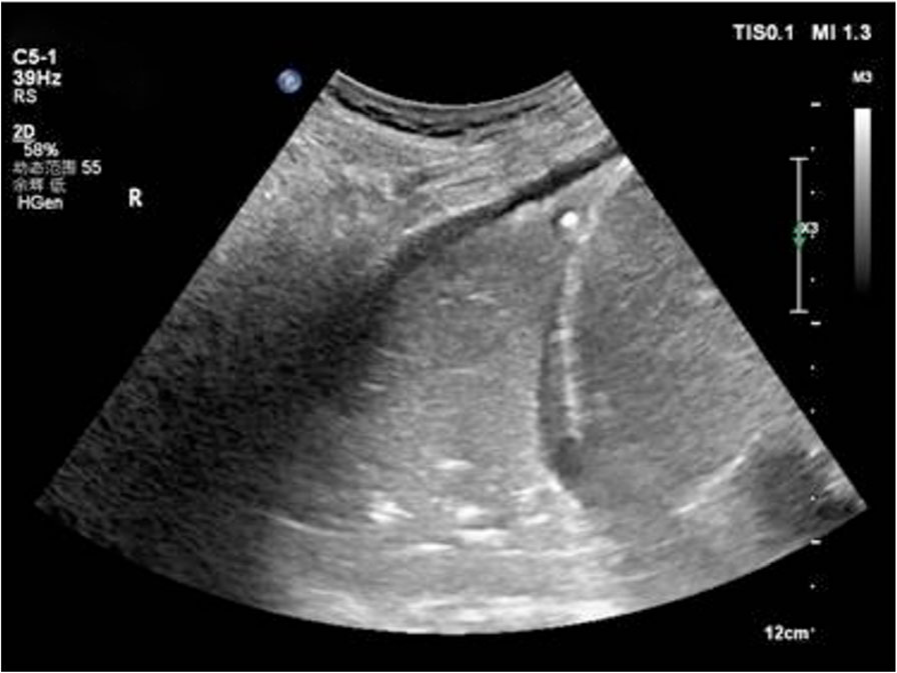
Lung consolidation: the sonographic sign of lung consolidation is a subpleural echo-poor region or one with tissue-like echotexture
